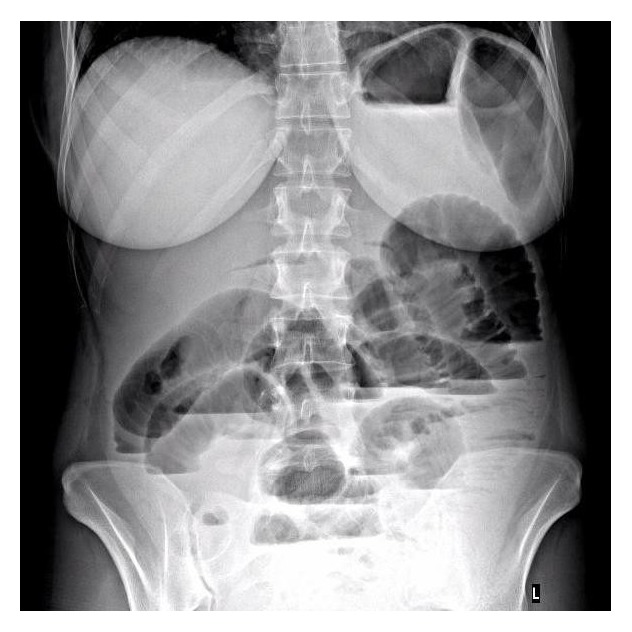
Erect abdominal X-ray on admission with distended bowels showing fluid levels.
